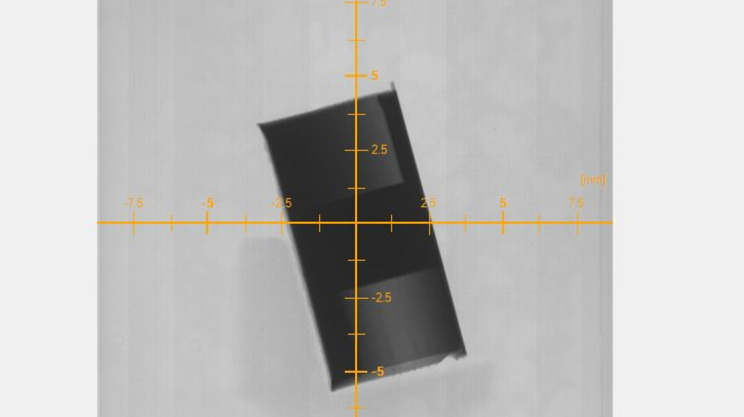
An example radiograph showing Part 1.
